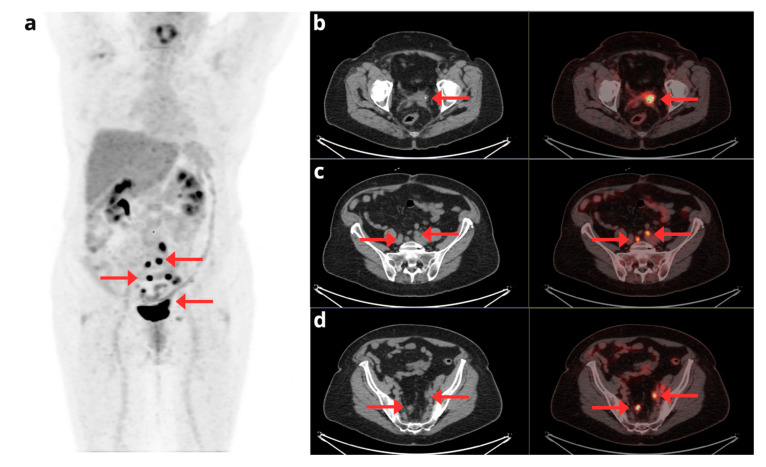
A 72-year-old woman with a history of stage IA G2 endometrioid EC, treated in October 2014 with laparoscopic hysterectomy and bilateral salpingo-oophorectomy. In May 2019, a follow-up CT scan revealed a suspicious nodular image suggestive of recurrence in the vaginal cuff, prompting a [^18^F]F-FDG PET/CT request in June 2019. The [^18^F]F-FDG PET/CT confirmed disease recurrence; the maximum intensity projection PET image (a) shows focal involvement in the vaginal cuff and pelvic lymph nodes (red arrows). Axial CT and PET/CT slices (b–d) localize the recurrence to the left-sided vaginal cuff (b) and additionally demonstrate metastatic lymphadenopathies in the common iliac chains (c) and bilateral internal iliac chains (d).
